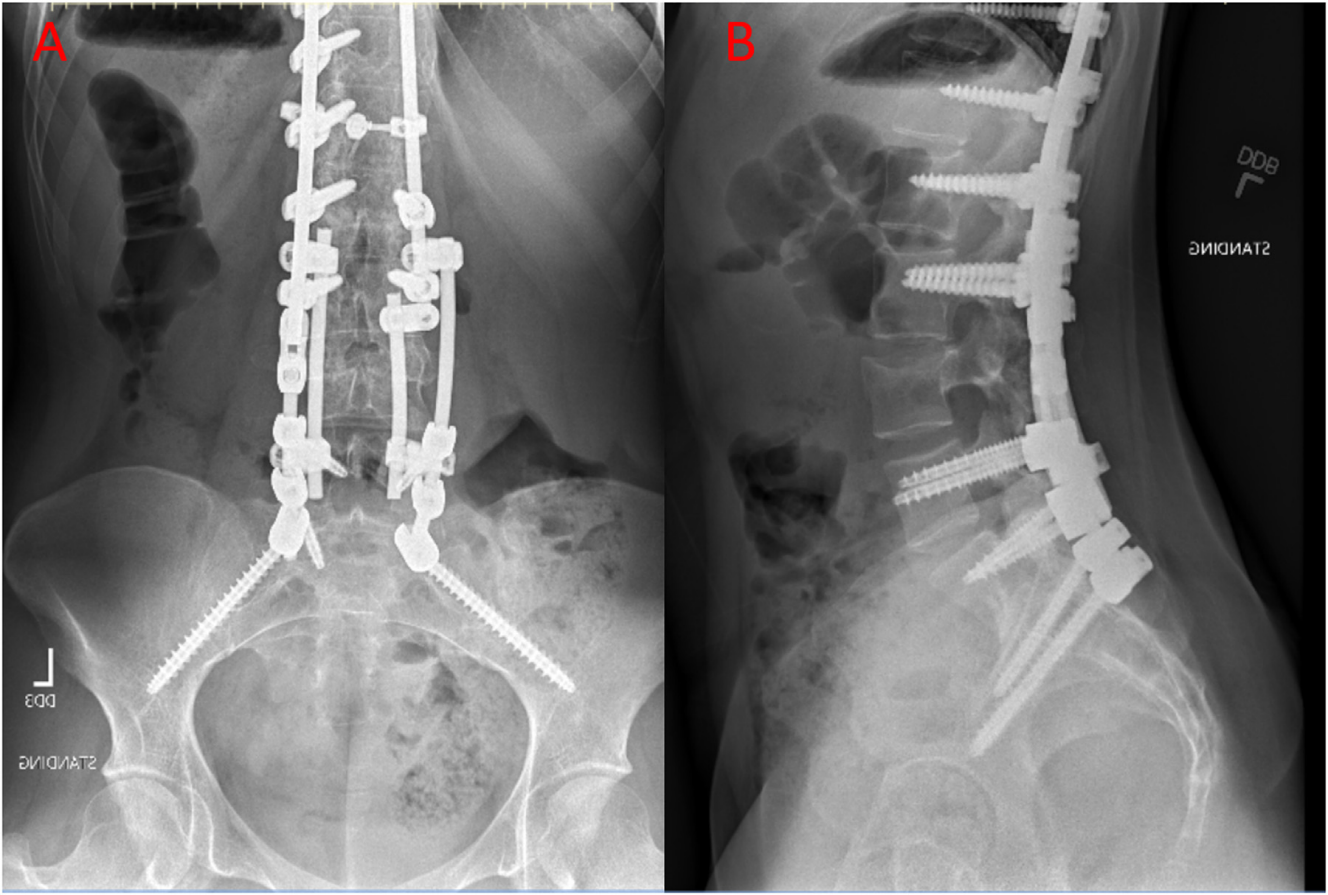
(A–B) Anteroposterior and lateral plain radiograph showing no implant related complication and a healed L4 vertebral body.
